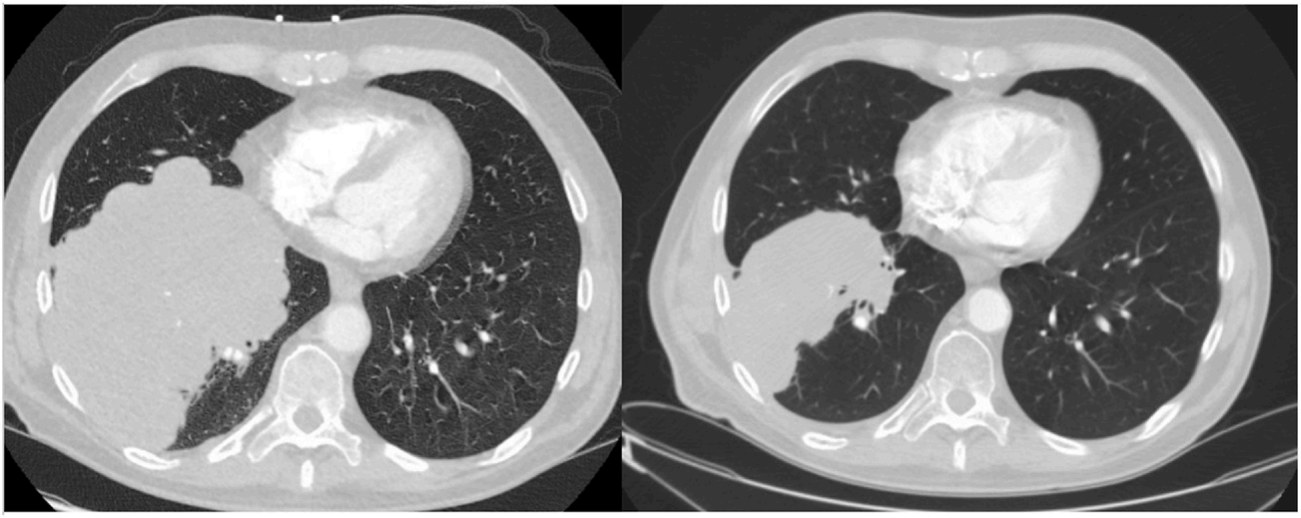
Chest computed tomography scan before and after four cycles of chemoradiation (A) Chest CT scan at diagnosis of combined SCLC revealed a 15.4 cm × 13.2 cm lobulated mass in the right lower lobe. (B) Repeat chest CT scan after four cycles of concurrent chemoradiation therapy showed decrease in size of the mass (10.5 cm × 6.3 cm).
